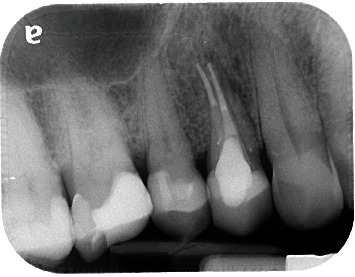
Radiograph of the upper right first premolar showing radicular radiolucency.
